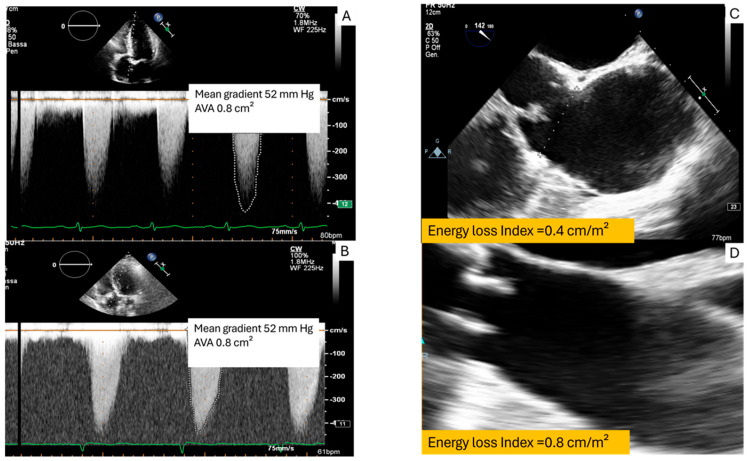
Echocardiographic assessment of severe aortic stenosis with a mean gradient of 52 mmHg and an AVA of 0.8 cm^2^. Depending on whether the ascending aorta was enlarged (C) or normal in size (D), the energy loss index (ELI) ranged from 0.4 to 0.8 cm^2^/m^2^, underscoring its role in refining severity classification beyond conventional Doppler indices. (A,B): Doppler transthoracic echocardiography in two different patients with the same mean gradient; (C,D): transesophageal echocardiography with different ascending aortic size.
